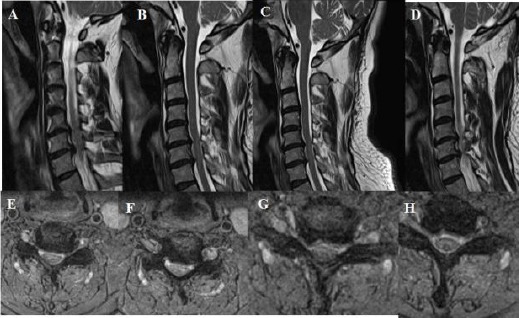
A, B, C, D, E, F, G, H: T2 weighted sagittal and axial MRI showing Compression to the medulla spinalis at two levels which are between cervical 5 and cervical 6, and between cervical 6 and cervical 7. Osteophyte formation and posterior longitudinal ligament ossification lead to this compression on medulla spinalis. Besides, cervical lordosis angle was measured 6.4 degrees on lateral X-ray at the preoperative period*.
